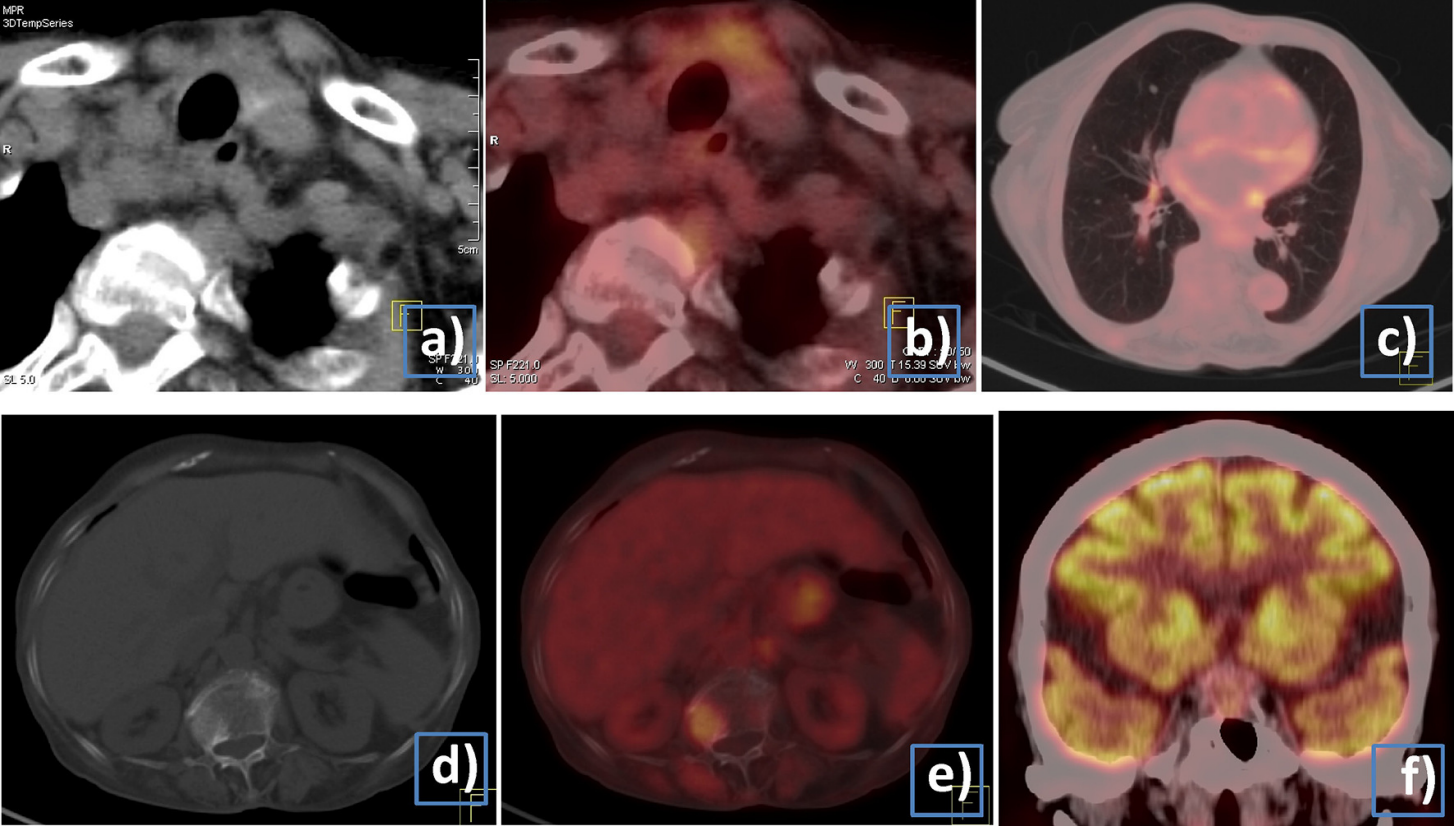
(a–f) ^18^F-FDG PET-CT. (a, b) CT and fused ^18^F-FDG PET-CT transaxial images showing residual thyroid tissue in the neck, predominantly on the left side; (c) fused ^18^F-FDG PET-CT transaxial image shows multiple small lung nodules; (d, e) CT and fused ^18^F-FDG PET-CT transaxial images show a sclerotic lesion in the vertebra with mild FDG uptake; (f) coronal ^18^F-FDG PET-CT fused image shows minimal FDG uptake in the sellar lesion. ^18^F-FDG PET-CT, 18-fludeoxyglucose positron emission tomography-CT.
